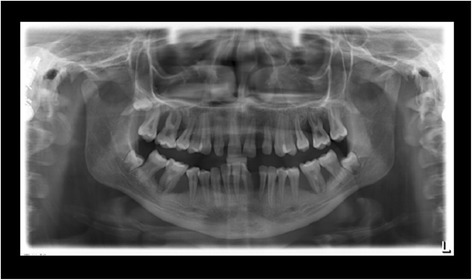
Panoramic radiograph at the age of 16 years: three years following conventional quadrantwise supra- and subgingival debridement without any supportive periodontal therapy.
